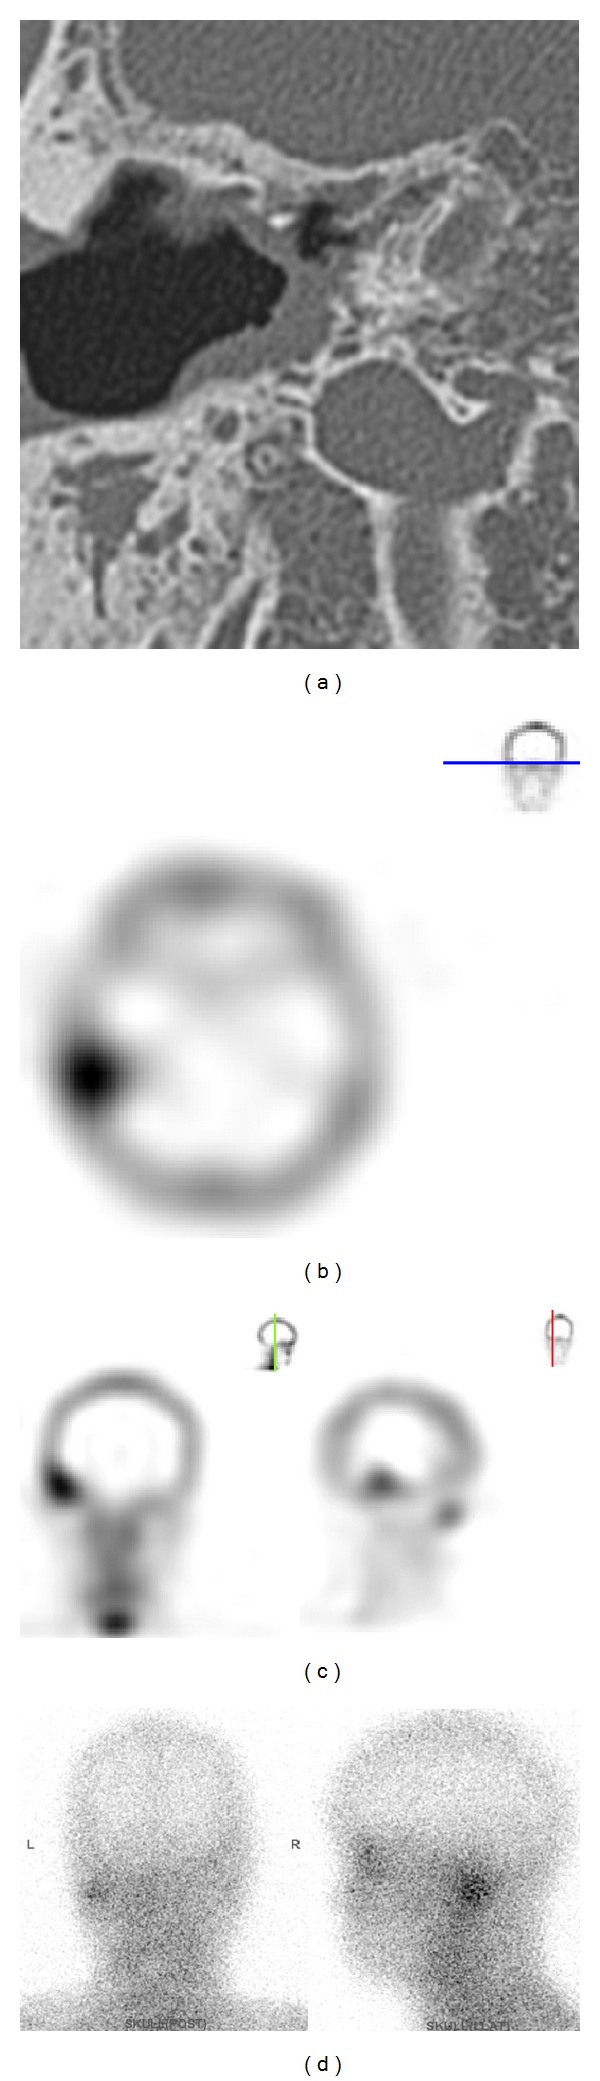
Images of a patient of temporal bone osteomyelitis after surgery for chronic otitis media with cholesteatoma. (a) CT. (b, c) Tc99m bone scan. (d) Gallium scan. See text for details.
